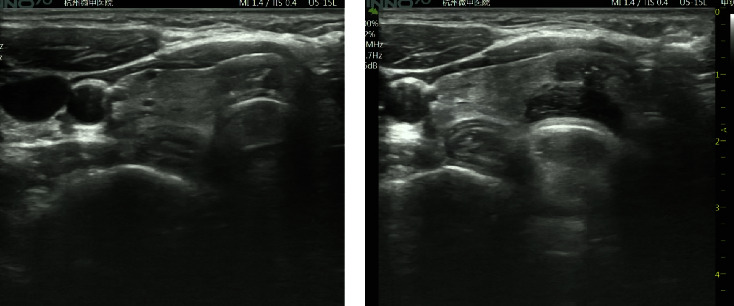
Ultrasound images before and after injection of hydrodissection: (a) image of the tumor before injection of hydrodissection and (b) image of the tumor after injection of hydrodissection.
